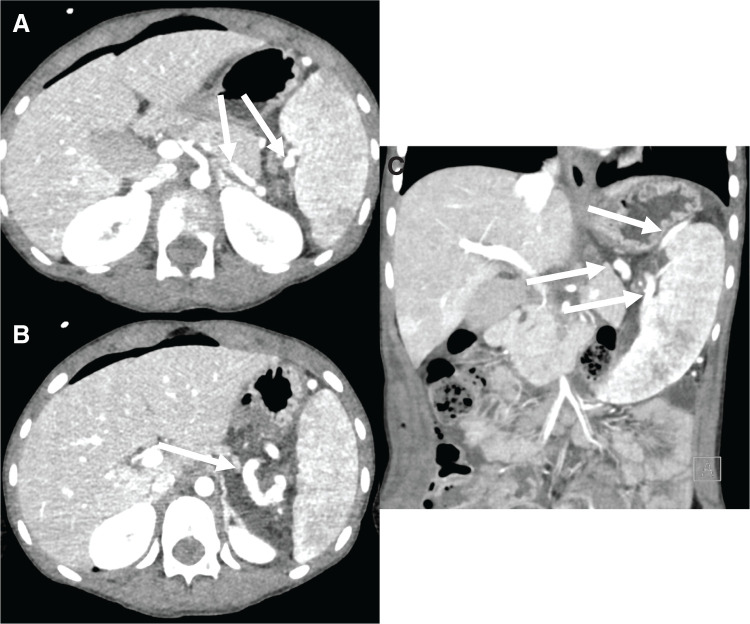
Abdominal CT scans taken on the day following detorsion (A) The blood flow of the main splenic vein was restored (white arrow). The axial (B) and coronal (C) images reveal enhanced spleen and collateral circulation from gastroepiplonic vein (white arrow).
